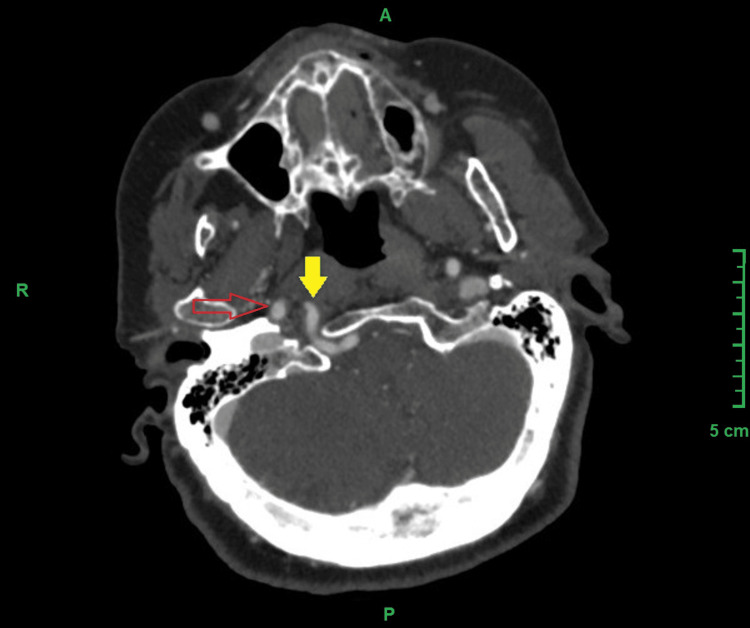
MSCT angiography, axial view, showing the persistent hypoglossal artery passing through the hypoglossal canal. Persistent hypoglossal artery, marked with a yellow arrow, and right internal carotid artery, marked with a red arrow. MSCT: Multi-slice computed tomography
